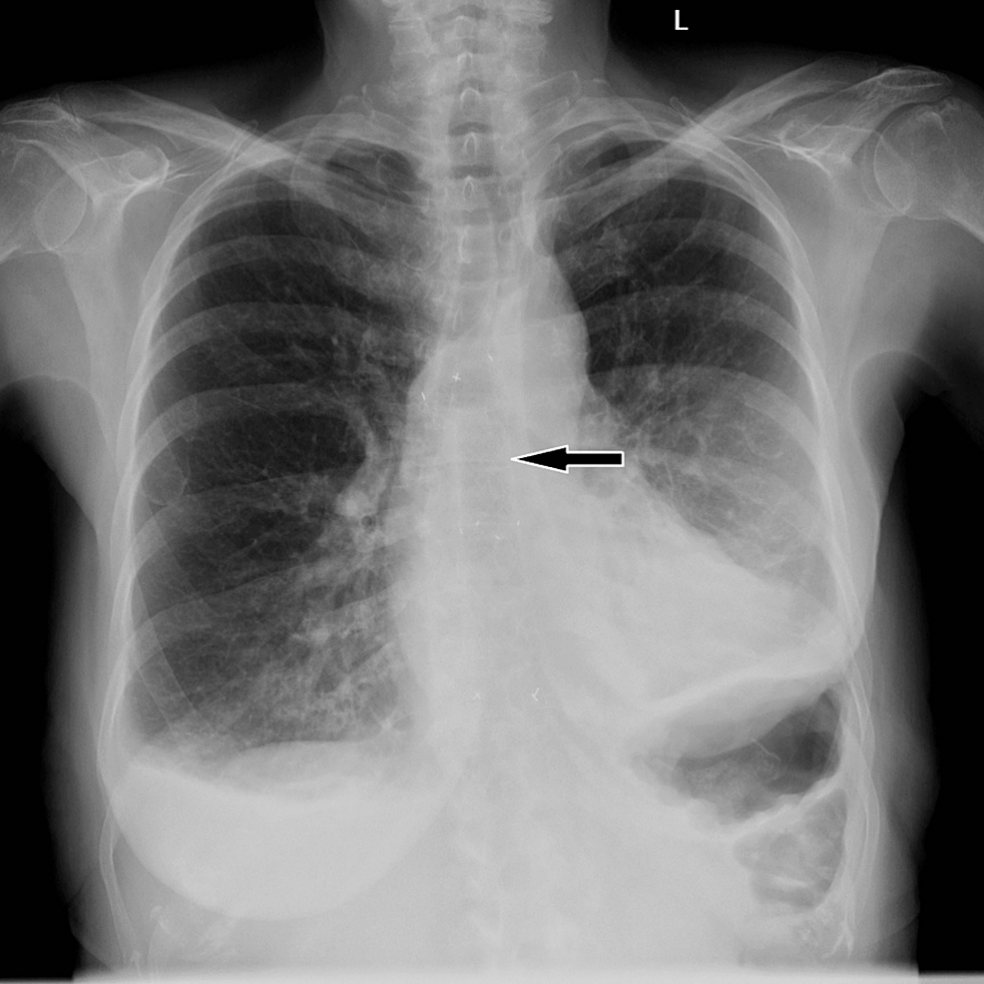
Chest radiograph after the initial esophageal stent placement (black arrow).
